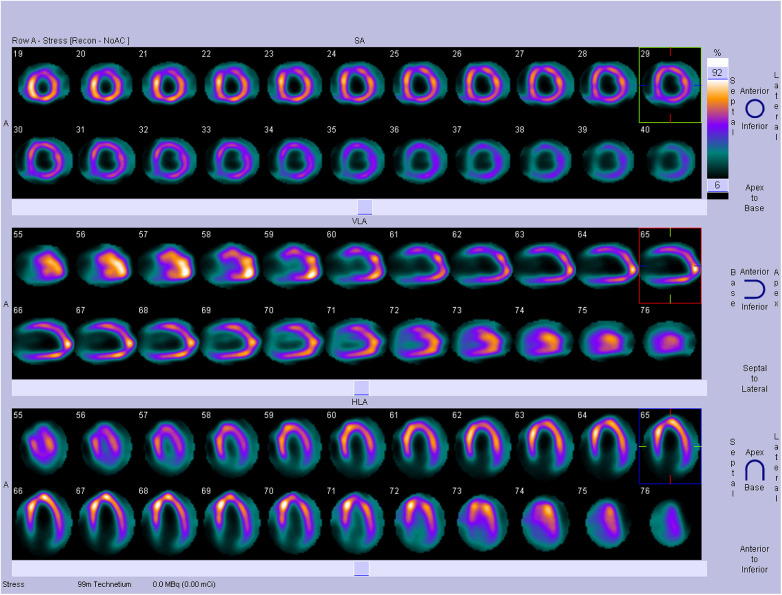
Single-photon emission computed tomography stress myocardial perfusion scintigraphy showing substantial hypoperfusion in the left ventricular anterior wall and anterior septum wall.
